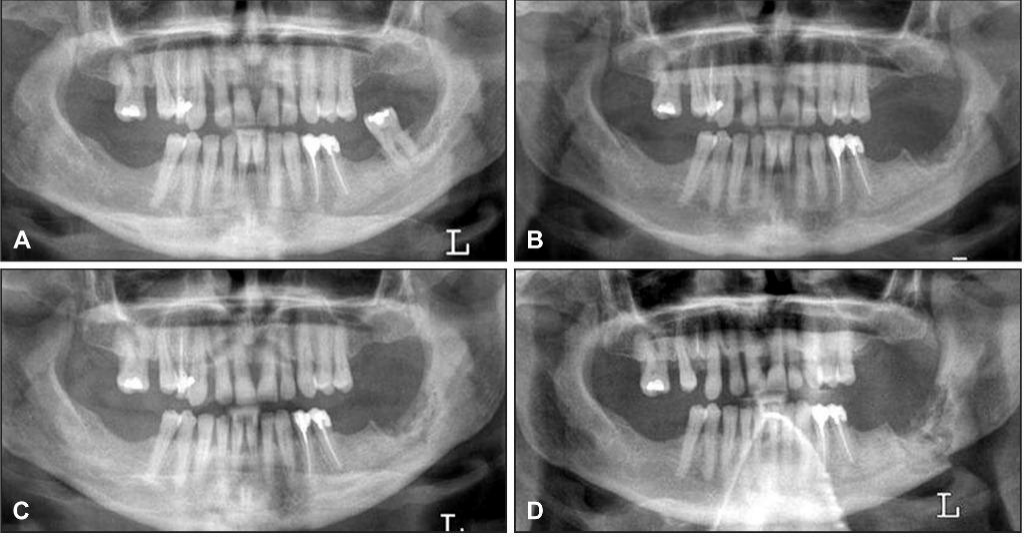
A = Panoramic radiograph of a woman with breast cancer treated with zoledronate ‎who presented with a painful tooth # 37‎‏.‏ B = Panoramic radiograph after 7 months, showing non healing extraction site in the left ‎posterior mandible, absence of bone remodeling and sclerotic bone changes of the body of the ‎mandible‏.‏ C = Panoramic radiograph after 9 months demonstrates a nonhealing extraction site in the left ‎posterior mandible with progressive sclerosis of the left body and angle of the mandible with ‎encroachment on the left mandibular canal‏.‏ D = Panoramic radiograph after 19 months with intervening curettage, demonstrates progression ‎of sclerosis to pathologic fracture of the mandible.
